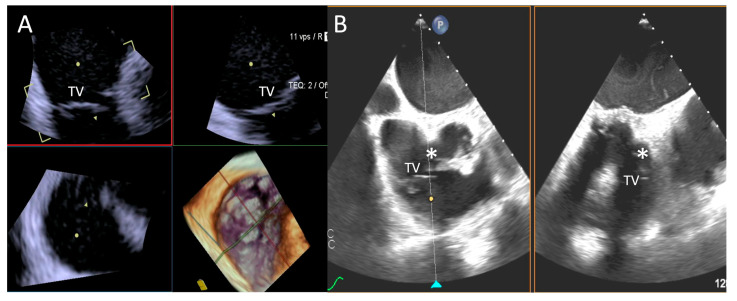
Step 1: insights from Case 4. The figure shows a comparative analysis of the tricuspid valve morphology evaluation during Step 1. In this case, the 4D-ICE (A) system shows better image quality in assessing TV morphology than TEE (B) due to anterior structures shadowing (*). Abbreviations: 4D-ICE: four-dimensional intracardiac echocardiography. TEE: transesophageal echocardiography. TV: tricuspid valve.
